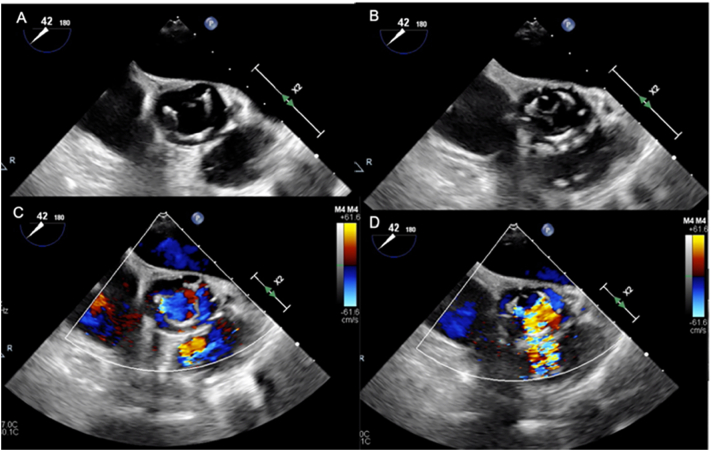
Transesophageal echocardiography. Short axis view; A: systolic phase, B: diastolic phase. Short axis view with color doppler; C: systolic phase, D: diastolic phase.
